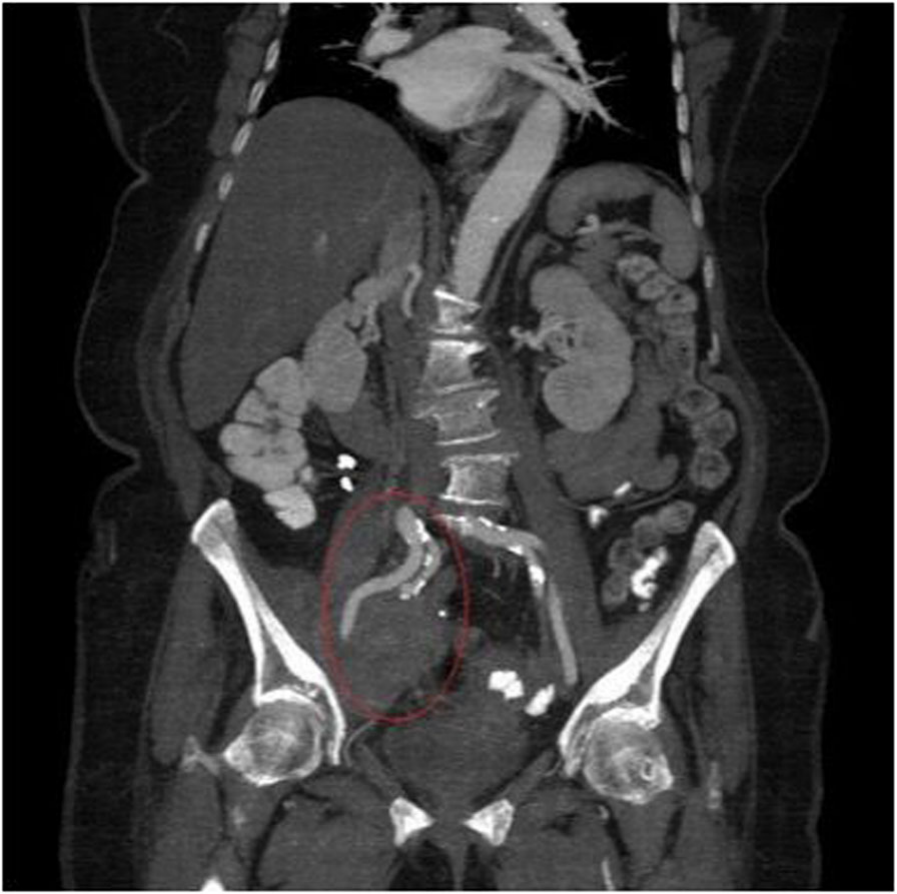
Frontal or coronal abdominal computed tomographic scan. The tumor lies on the external iliac artery (encircled)
